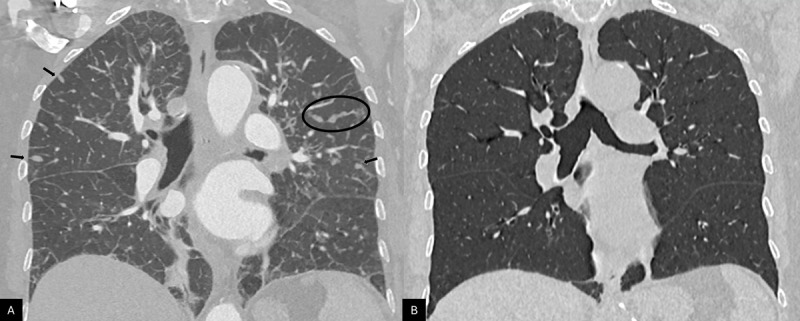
Comparison between a coronal CT view obtained on the day of admission (A) and a coronal CT view performed eight weeks later (B), showing multiple diffuse ovoid and branched nodules (black arrows) distributed along thickened interlobular septa (black circle), which resolved on follow‑up imaging.
